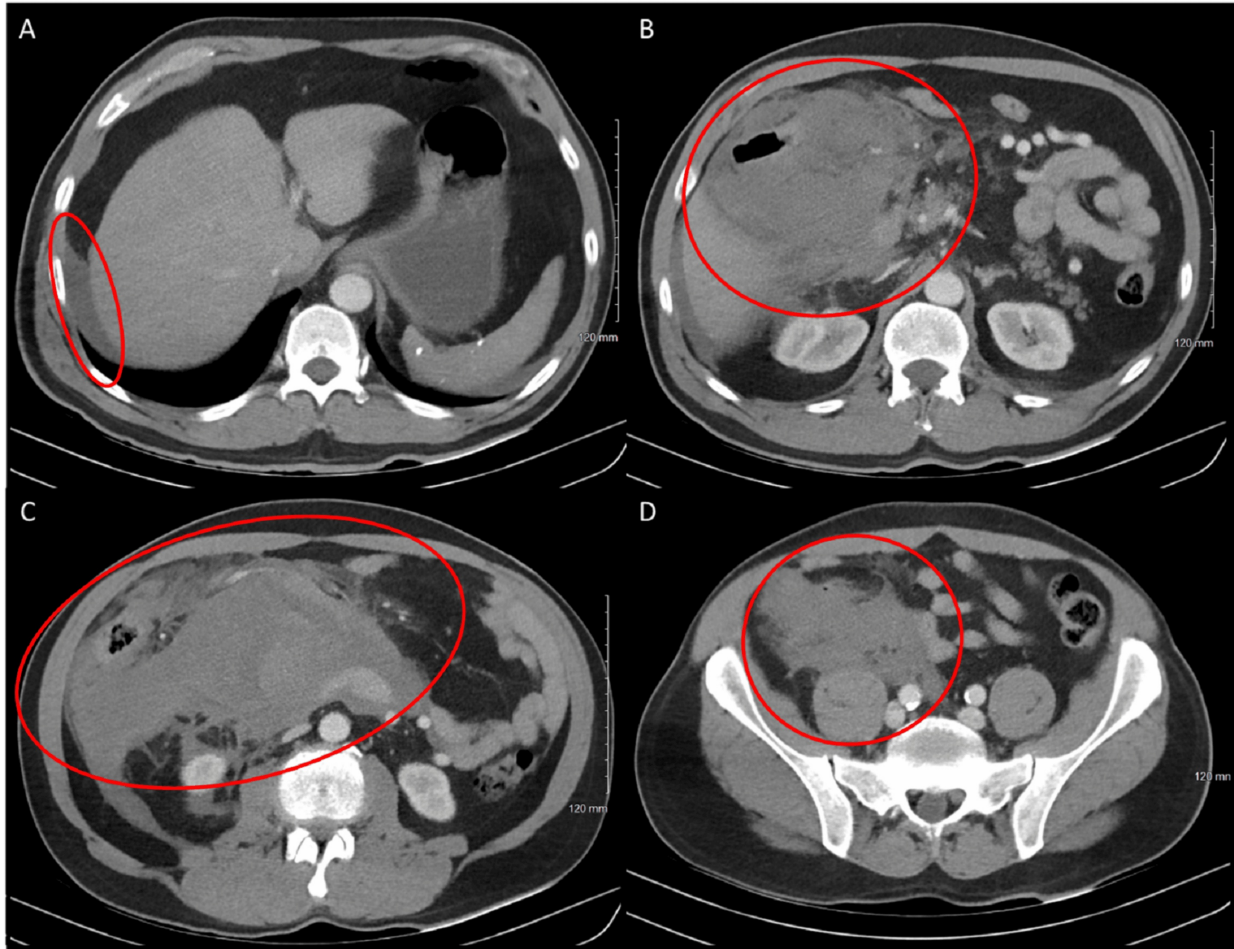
Axial cuts through a CT abdomen pelvis with intravenous contrast, demonstrating free fluid within the abdomen. (A) Free fluid along the right lateral edge of the liver (red circle). (B) Free fluid in the mid-upper abdomen at the inferior edge of the liver (red circle). (C) Free fluid in the lower abdomen (red circle). (D) Free fluid within the right hemipelvis (red circle) CT: computed tomography
